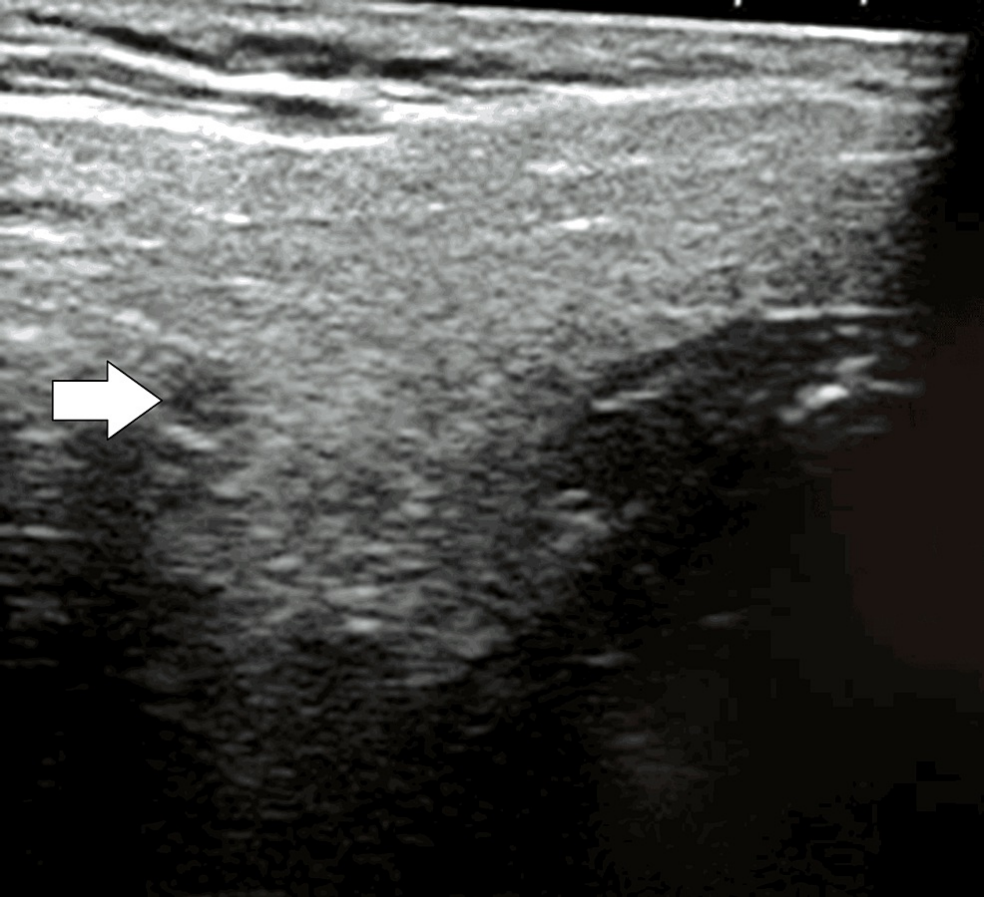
Ultrasonography showing a bulky parotid without any rise in vascularity
